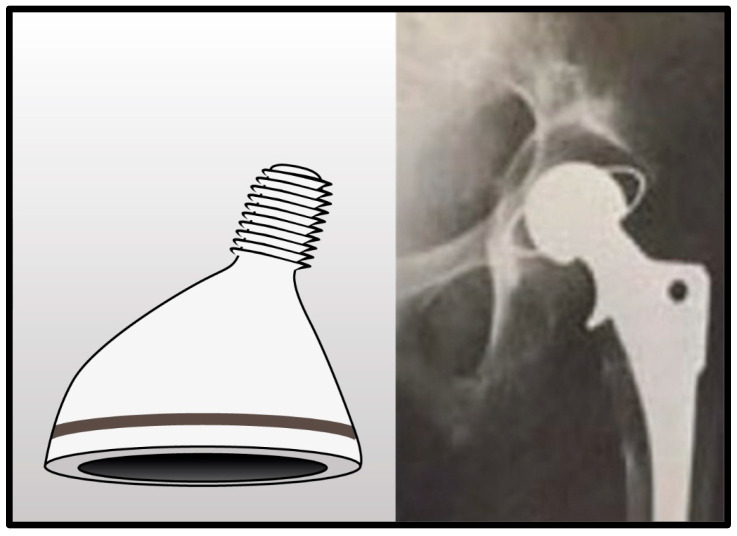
All-polyethylene acetabular component with a 32 mm femoral head and angled peg impacted into bone. Radiographic image demonstrates component failure associated with significant osteolysis.
